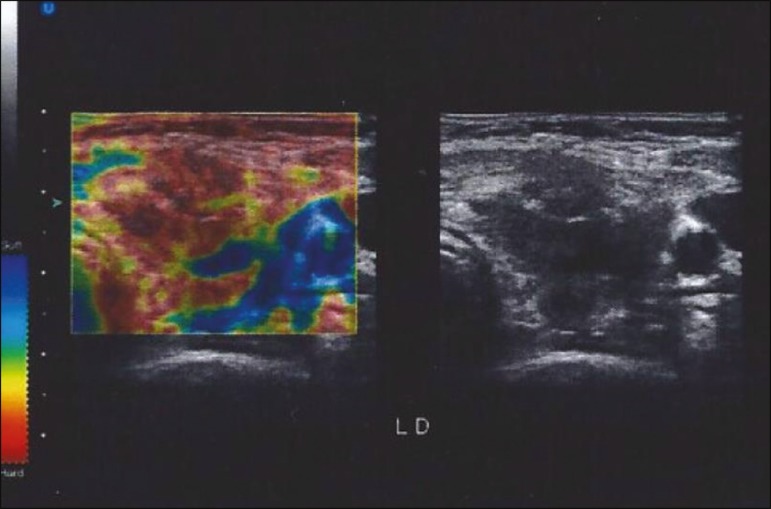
Green light appears to the left of the ultrasound image when the degree
